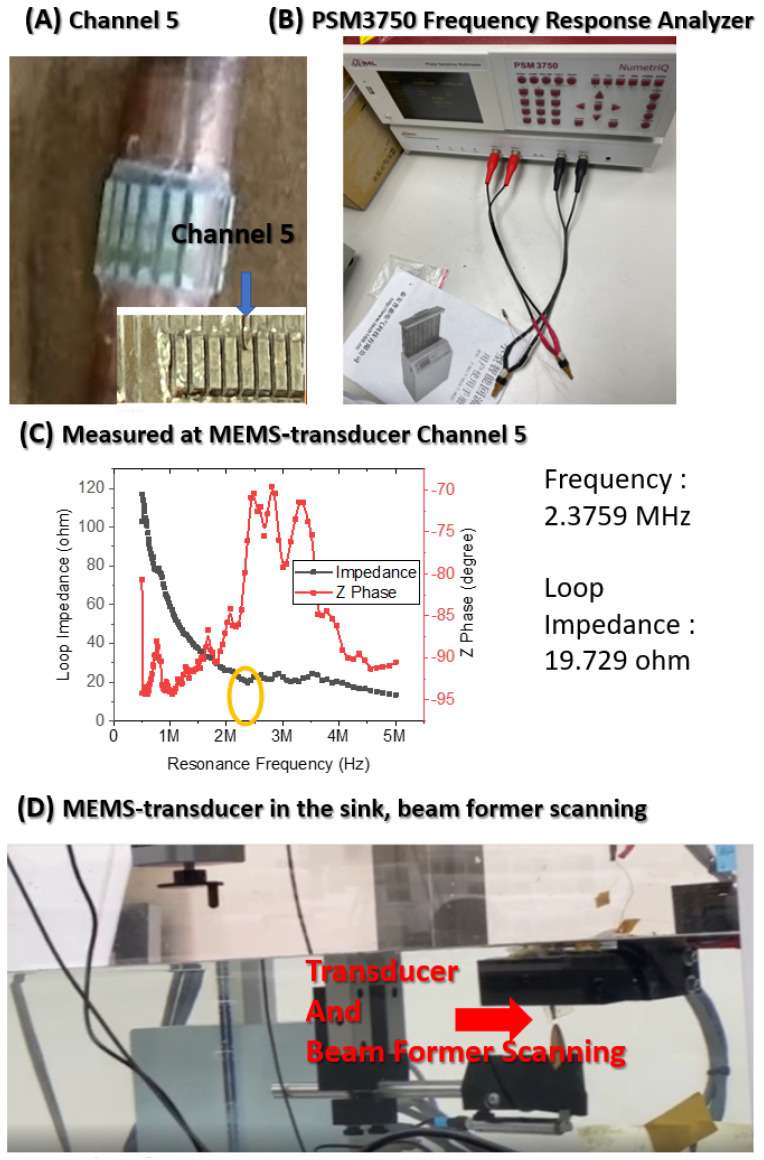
Channel 5 generated by ultrasonic energy was selected to increase the voltage supply. (A) The 16-channel circular MEMS-transducer array; (B) The PSM3750 Frequency Response Analyzer (Miko-Kings Instruments LTD, Fotan, Shatin, N.T, Hong Kong) was used for measuring the resonance frequency of the piezoelectric element; (C) Measured at MEMS-transducer Channel 5, the frequency and impedance at the resonance frequency were 2.3759 MHz and 19.729 Ω, respectively; (D) After putting the MEMS-transducer in the sink, beamforming was performed to scan its ultrasonic resonance energy.
